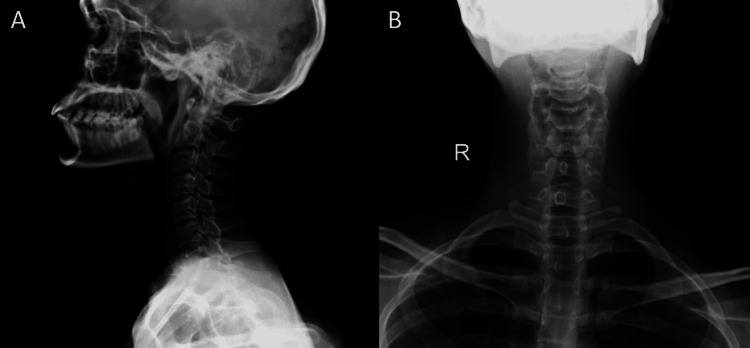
X-ray of the cervical spine lateral (A) and anteroposterior (B) view revealed no abnormality.
